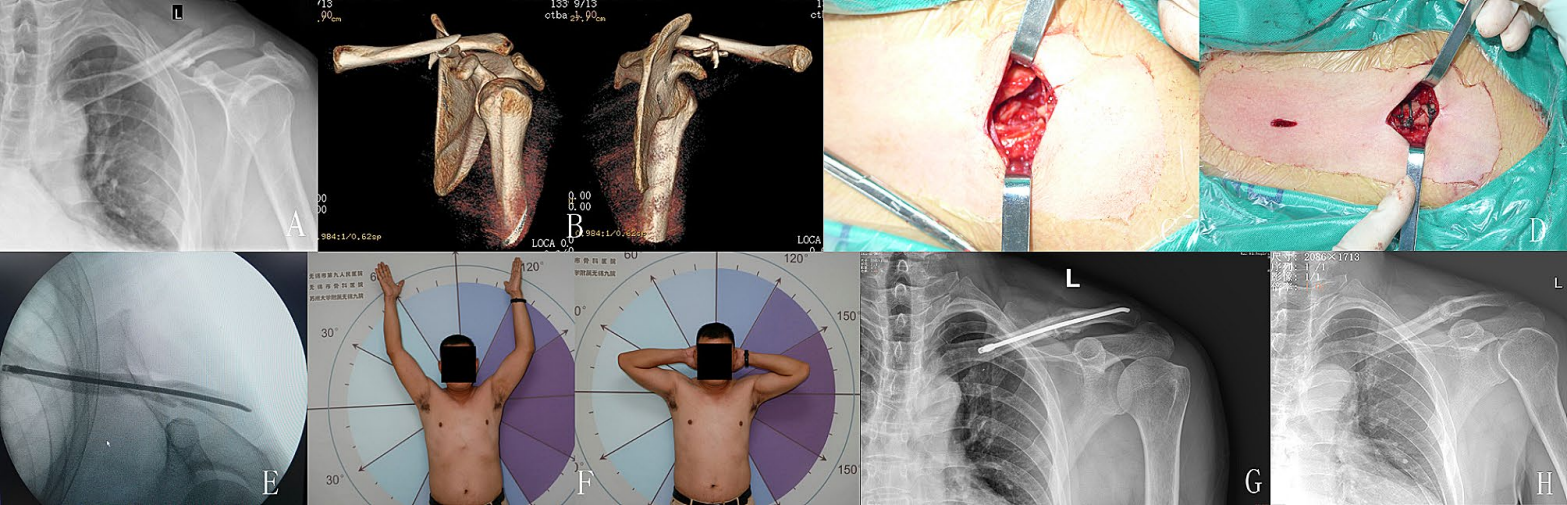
A 41-year-old man had a traffic injury, resulting in a Robinson 2B2 left midshaft clavicle fracture. A/B: Radiography and CT of the fracture. C: Intraoperative fracture displacement. D: The fracture was reduced, and no. 2 Ethibond non-absorbable thread was used to fix the fracture with the Nice knot. E: Follow-up radiography of the fracture after surgery. F: Clinical appearance at 8 months after surgery. G/H: Radiography confirms fracture healing at 8 months after surgery, and the implant was removed
